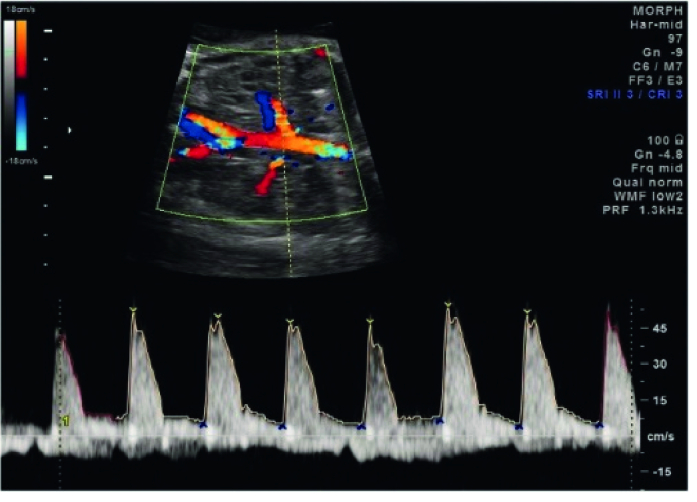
Fetal renal artery doppler.
